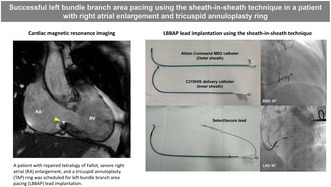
A 55‐year‐old woman was admitted to our hospital with sinus node dysfunction and presyncope symptoms (Figure 1A,B). She was diagnosed with tetralogy of Fallot at birth and underwent transannular patch repair at 4 years of age. At 49 years of age, she underwent two sessions of radiofrequency catheter ablation for atrial tachycardia originating from the right atrium (RA). Electroanatomical three‐dimensional mapping during the procedure revealed severe RA dilatation and extensive low‐voltage areas. During follow‐up, she developed severe tricuspid regurgitation and right ventricular (RV) enlargement. At 53 years of age, she underwent pulmonary valve replacement using an Inspiris 25‐mm valve (Edwards Lifesciences, Irvine, CA, USA) and tricuspid annuloplasty (TAP) with a Tri‐Ad Adams 30‐mm ring (Medtronic, Minneapolis, MN, USA). Cardiac magnetic resonance imaging performed prior to pacemaker implantation revealed enlargement of the RA and RV, as well as a surgically constricted tricuspid annulus with the TAP ring in place (Figure 1C). The left ventricular ejection fraction (LVEF) was 50%. In accordance with the current guideline‐directed therapy, transvenous dual‐chamber pacemaker implantation was scheduled to address symptomatic sinus node dysfunction [1]. Although the indication for left bundle branch area pacing (LBBAP) in patients with sinus node dysfunction and preserved LVEF remains controversial [2], informed consent was obtained from the patient for the implantation of a ventricular lead in the left bundle branch area, based on the following considerations: the presence of extensive low‐voltage areas in the RA suggested a potentially high risk of developing atrioventricular block due to intra‐atrial conduction disturbances and refractory atrial arrhythmias requiring atrioventricular nodal ablation.
